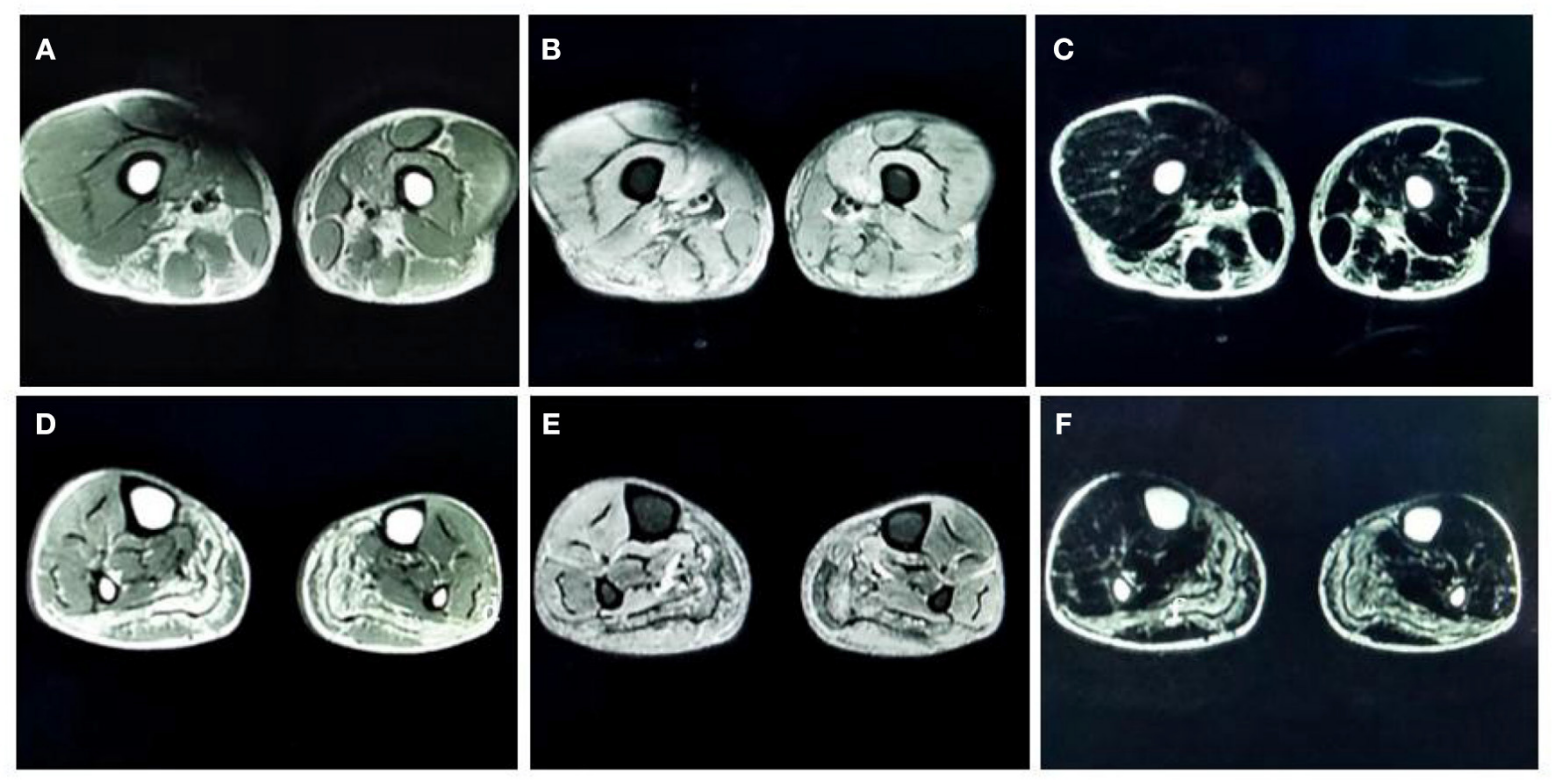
(A) T1-weighted (T1-WI) magnetic resonance imaging (MRI) of both thighs showed high signal intensities in the adductor magnus, biceps femoris, semitendinosus, and semimembranosus muscles. (B) Fat-suppressed T2-weighted (T2WI) images of both thighs showed high signal intensities in the vastus medialis and intermedius. (C) Water-suppressed T2WI MRI images of both thighs showed high signal intensities in the adductor magnus, biceps femoris, semitendinosus, and semimembranosus muscles. (D) T1-WI images of both lower legs showed diffuse high signal intensities in the medial and lateral heads of the gastrocnemius muscle and part of the soleus muscle. (E) Fat-suppressed T2WI images of both lower legs showed slightly higher signal intensities in the gastrocnemius muscle and part of the soleus muscles. (F) Water-suppressed T2WI MRI images of both lower legs showed high signal intensities in the gastrocnemius muscle and part of the soleus muscles.
